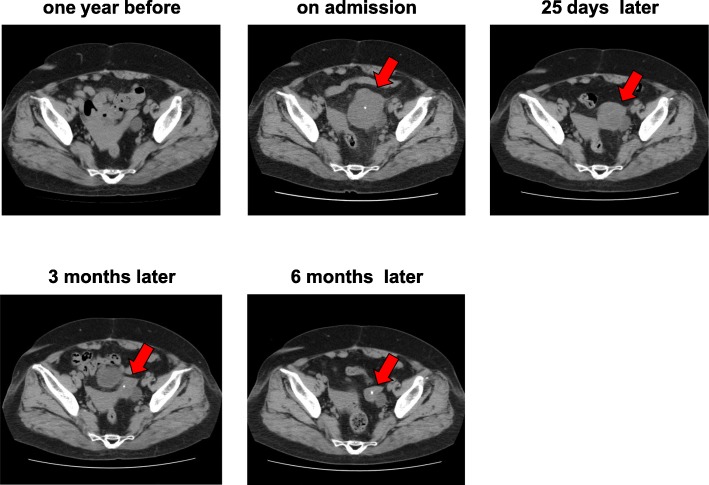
Abdominal computed tomography (CT) one year before (*upper left panel*), on admission (*upper middle panel*), 25 days later (*upper right panel*), 3 months later (*lower left panel*) and 6 months later (*lower middle panel*). Abnormal CT on admission showed a large tumor with calcification in the pelvis which was observed in abdominal CT one year before. Its size is as large as 65 mm in diameter (*upper middle panel*). Its tumor size was gradually reduced 25 days later (*upper right panel*), 3 months later (*lower left panel*) and 6 months later (*lower middle panel*) compared to that on admission (*upper middle panel*), but the tumor did not disappear completely even 6 months later. The arrows show the tumor which reduced in size at time course
